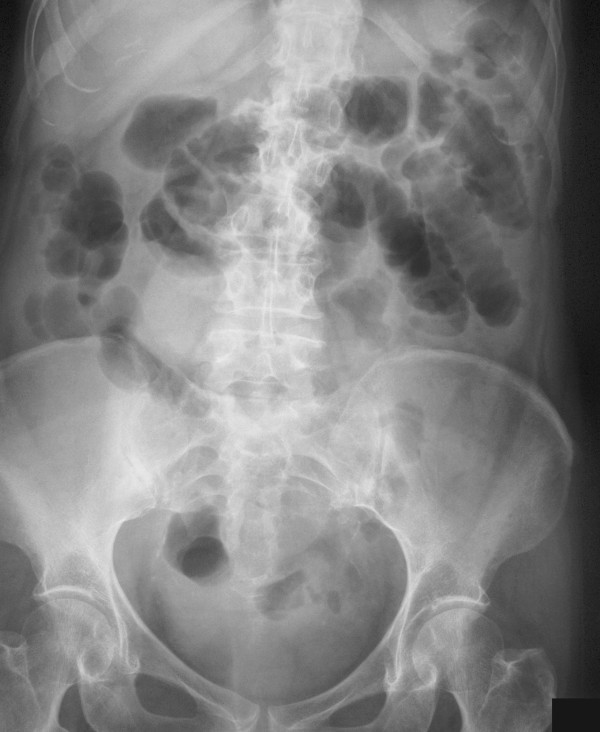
Plain abdominal radiograph. This radiograph was captured 16 hours after colonoscopy. Several loops of dilated and oedematous small bowel are visible in the left upper quadrant of the abdomen.
